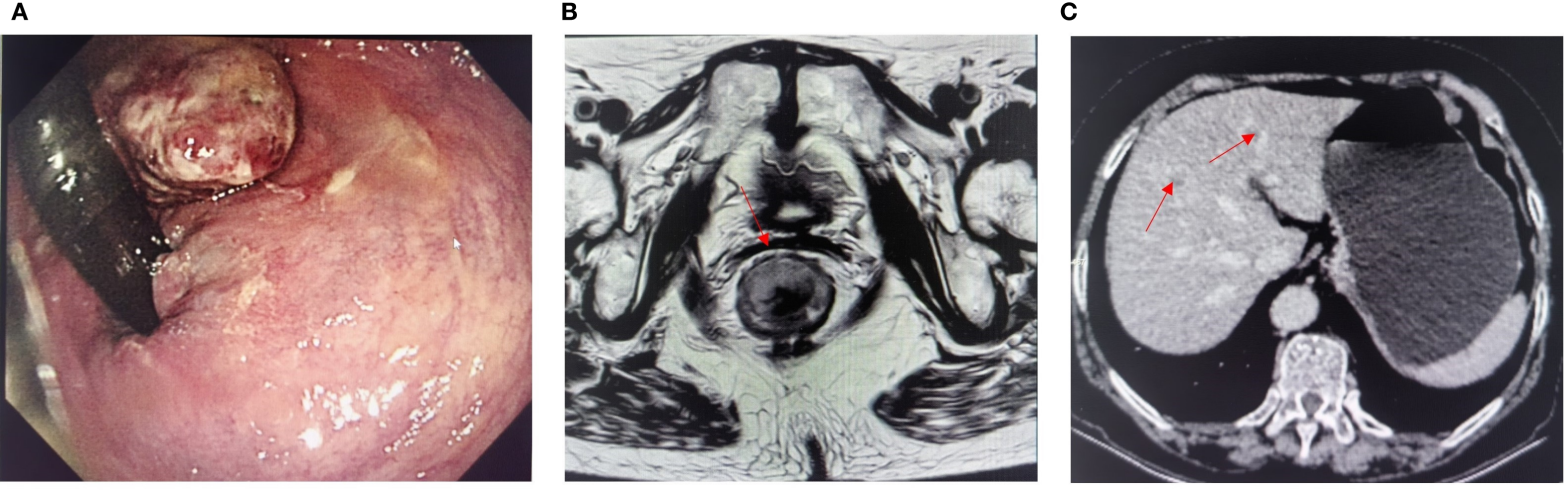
(A) Preoperative colonoscopy image. (B) Magnetic resonance imaging evaluation before surgery. (C) Enhanced CT showed liver metastases (red arrows indicate lesions).
